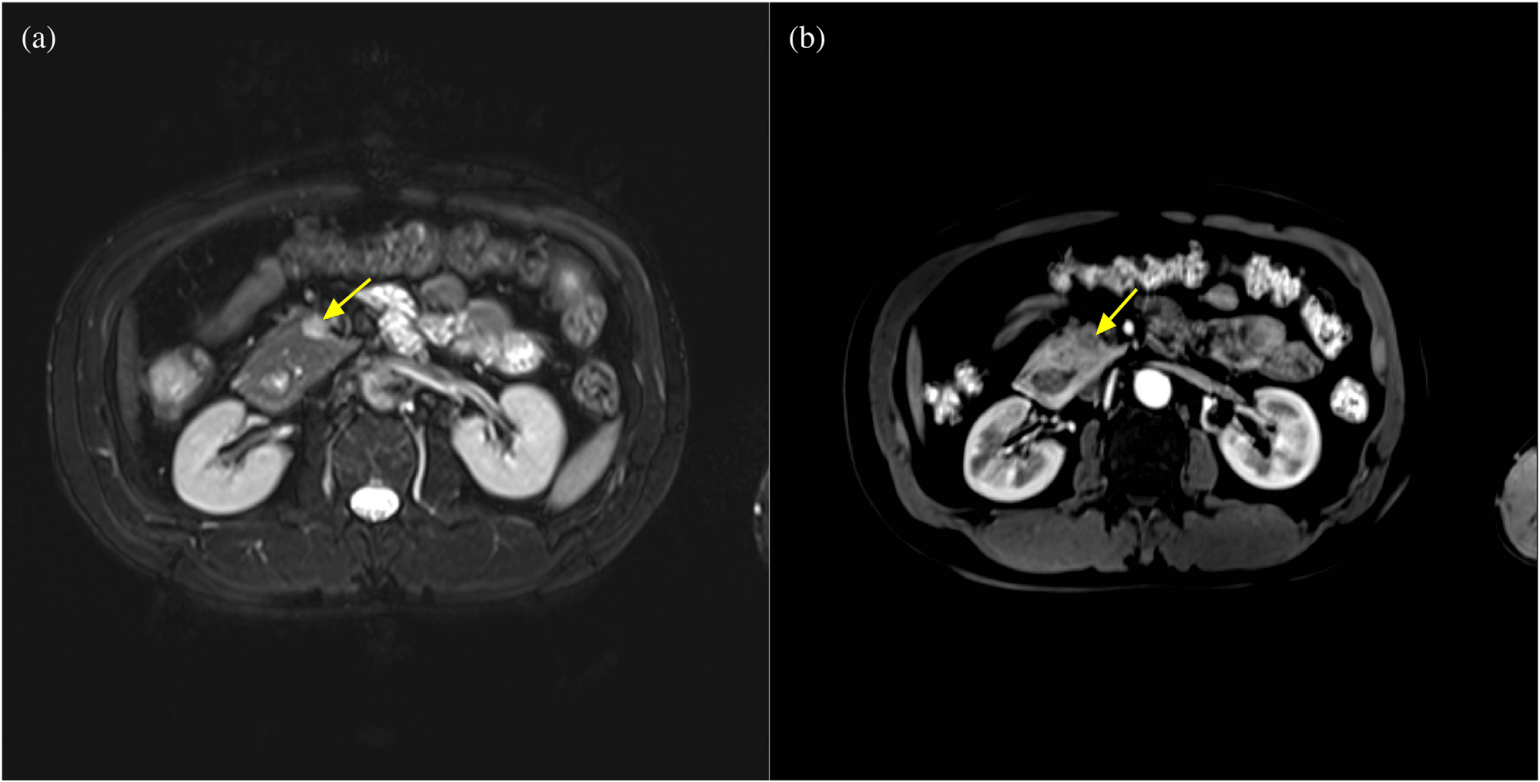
A 45‐year‐old man with annular pancreas and insulinoma. (a) Axial fat suppressed T2‐weighted magnetic resonance (MR) image shows neoplasm (arrow) in the pancreatic head which manifests as hyperintensity. (b) Axial fat suppressed T1‐volumetric interpolated breath‐hold examination (VIBE) arterial phase MR image shows that the neoplasm is slightly enhanced
